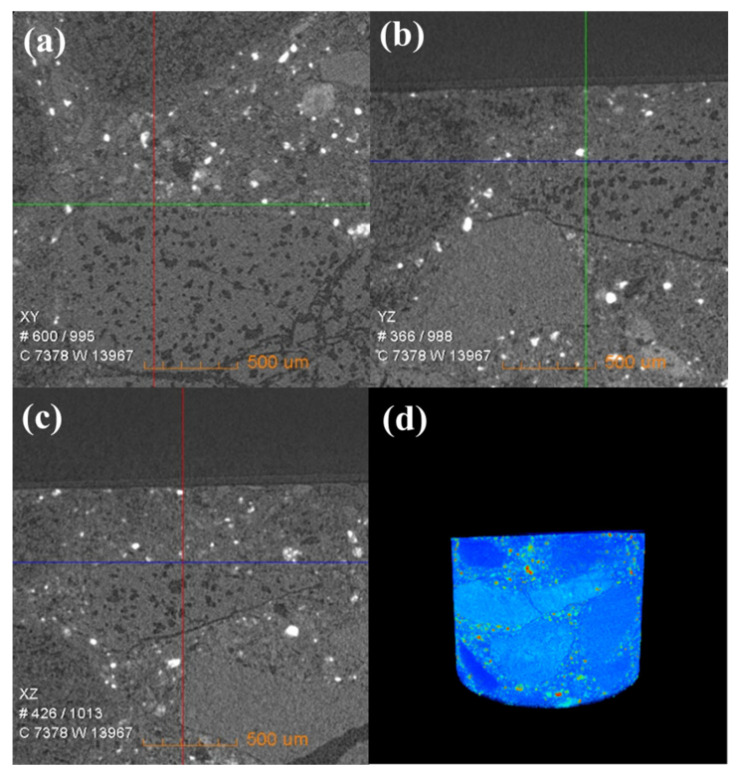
X-ray CT of a new saggar sample. (a), (b), and (c) Internal X-ray CT images of a new saggar sample scanned from the XY, YZ, and XZ directions, respectively. (d) Three-dimensional image in which bright and dark colors represent high and low density, respectively.
